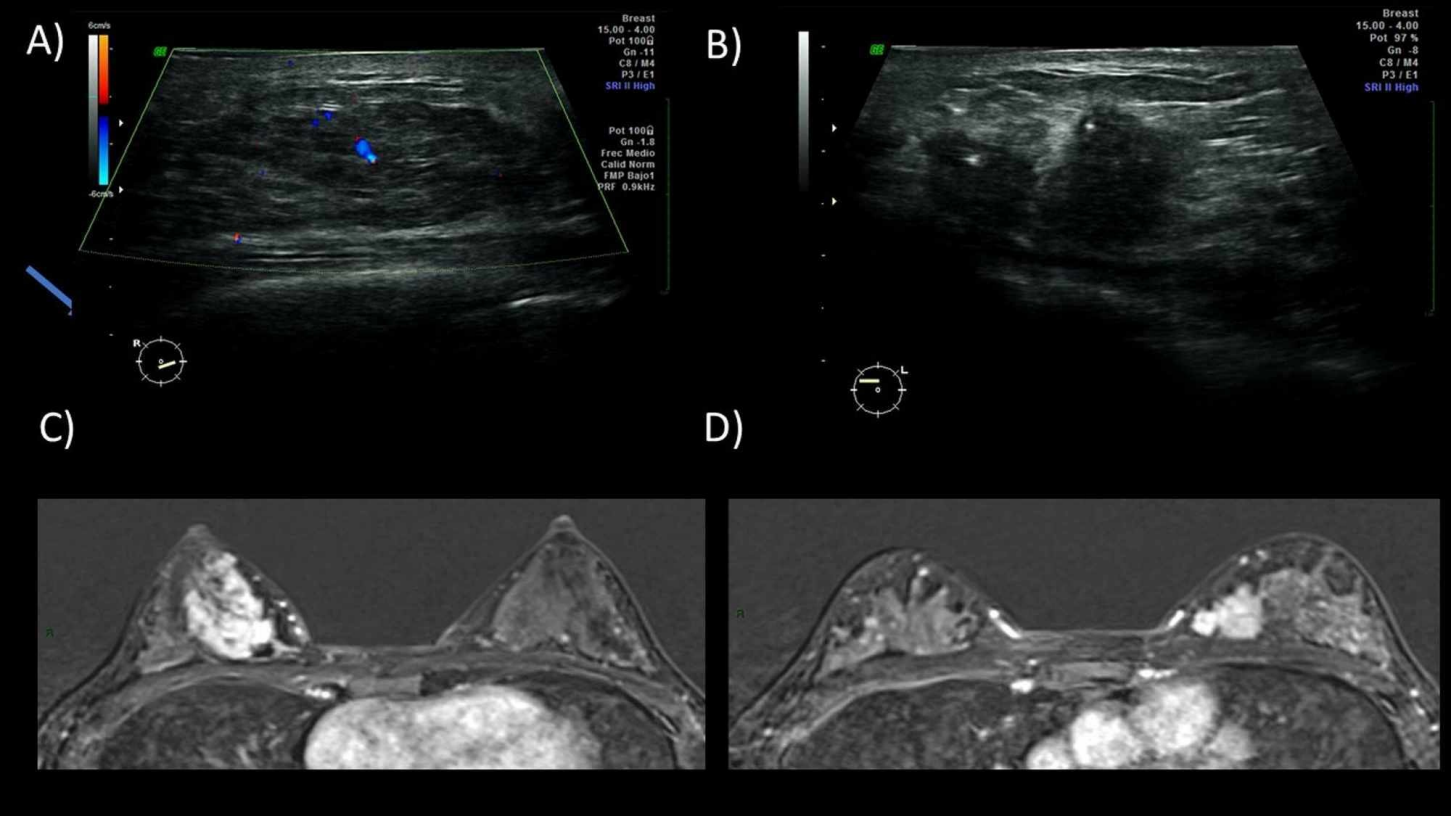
A 38-year-old female patient in puerperium and lactation felt nodules on both breasts since her first trimester. Ultrasound was performed. (A) The right breast showing an isoechoic nodule with ill-defined borders with central vascularity. (B) The left breast showing a hypoechoic nodule with irregular margins and no echogenic pseudocapsule. Bilateral MRI (C and D) was performed with bilateral masses showing enhancement and restriction in the diffusion sequence (not shown). A bilateral percutaneous biopsy was performed with typical hyperplasia on the right breast and infiltrating lobular carcinoma on the left breast.
